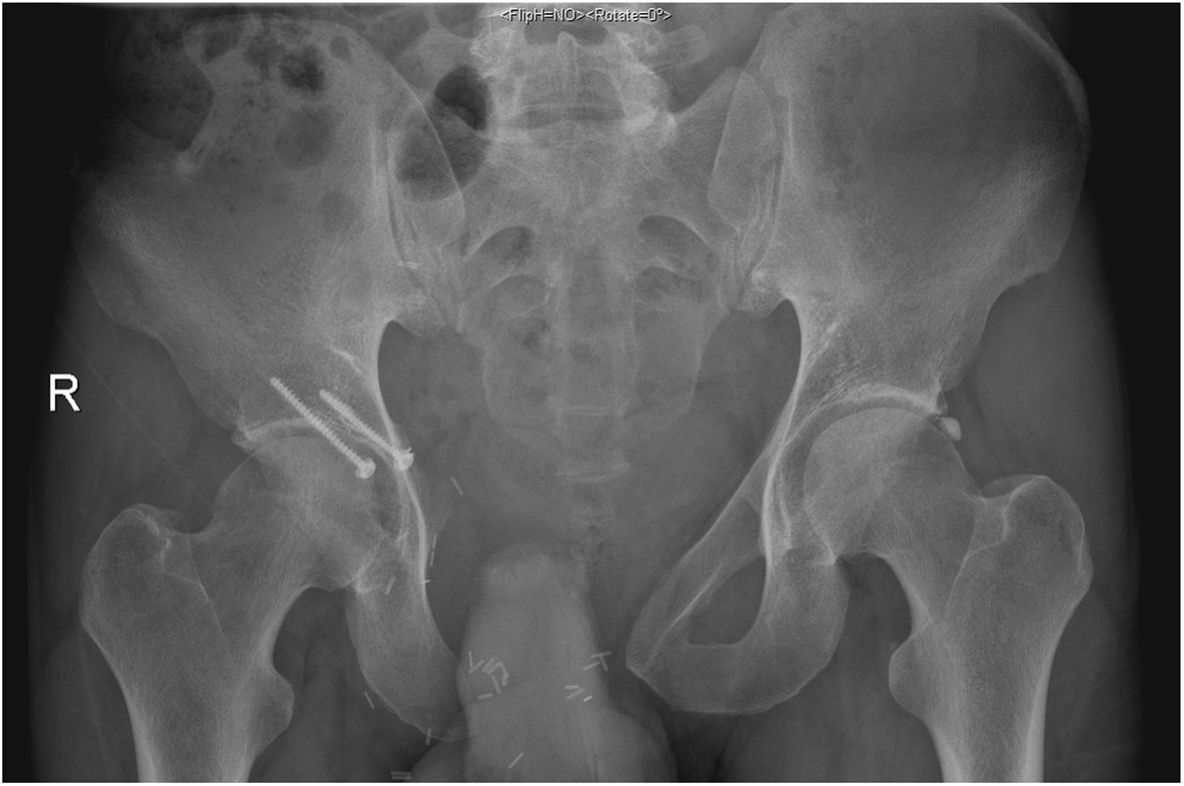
Plain radiograph of a pelvis a.p. after partial type 3 resection of the pubic bone. A partial acetabular defect was stabilized using an autologous iliac crest graft osteosynthesis. The donor site is visible on the right iliac crest
